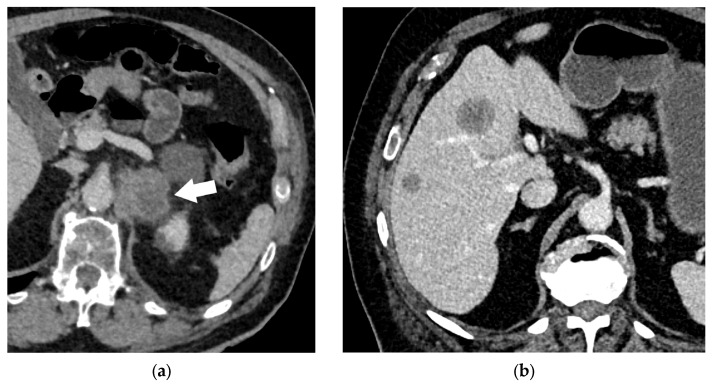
M1c2 category: Axial contrast-enhanced CT scan showing a strongly enhancing, heterogeneous, ill-defined mass in the left adrenal gland, indicative of a metastatic lesion (a). Multiple hypoattenuating liver lesions in portal venous phase CT suggest hepatic metastases (b).
